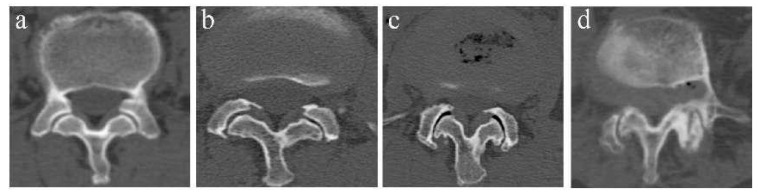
Example of FJOA on CT. (a) Grade 0: normal facet joint. (b) Grade 1: mild osteoarthritis facet joint with narrow space and small osteophytes. (c) Grade 2: moderate osteoarthritis with sclerosis or subchondral erosions. (d) Grade 3: severe osteoarthritis with marked osteophyte.
